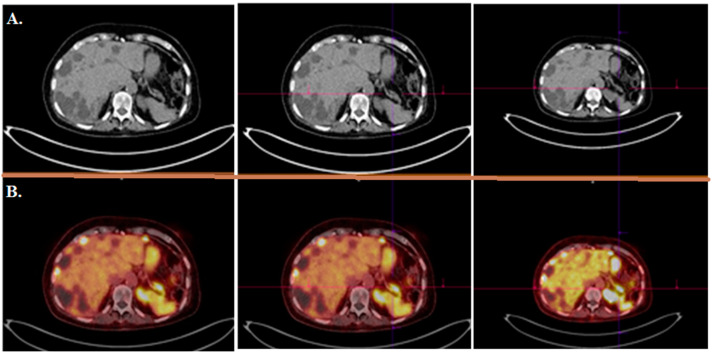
[^64^Cu]Cu-DOTA-TOC PET-CT: A 76-year-old gentlewoman with a history of resected neuro endocrine tumor of the appendix 10 years ago, with recurrence at multiple bilobar multisegmental liver metastases (image B); the primary tumor was detected as a suspicious lesion in the tail of the atrophic pancreas (image A); Ki67 9%.
